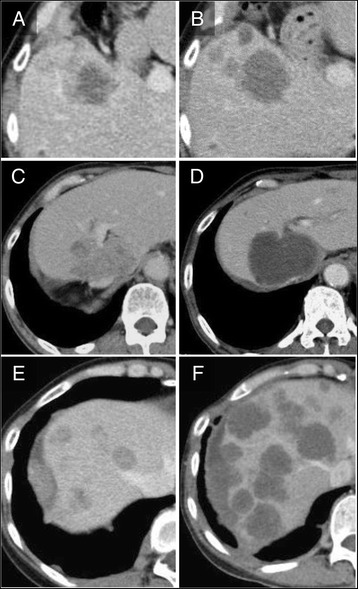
Patients presenting optimal morphologic responses during treatment with regorafenib. A, B. Optimal morphologic response observed at 175 days after initiation of regrafenib. Size of tumor was slightly increased (+ 6.0%) with reduction in CT density. CEA levels were 911 ng/ml (A) and 840 ng/ml (B), respectively. C, D. Optimal morphologic response observed at 105 days after initiation of regrafenib. Size of tumor was slightly increased (+ 16.0%) with evident change in CT texture of tumor. CEA levels were 860 ng/ml (C) and 910 ng/ml (D), respectively. E, F. Optimal morphologic response observed at 90 days after initiation of regrafenib. Although there was remarkable progression of tumor in number and size, serum CEA levels decreased from 91.7 ng/ml (E) to 66.1 ng/ml (F)
